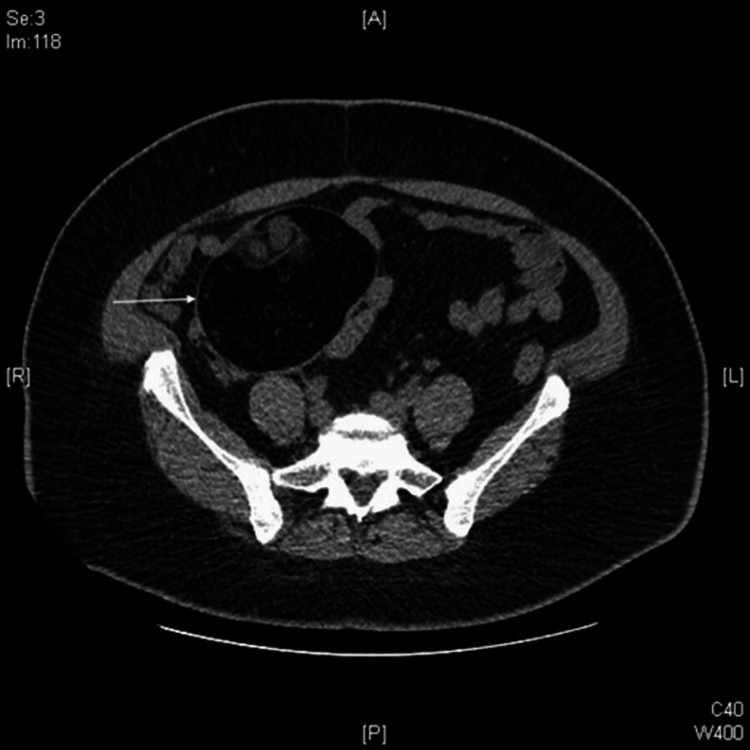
Abdominal and pelvic CT Arrow denotes ovarian mass.
